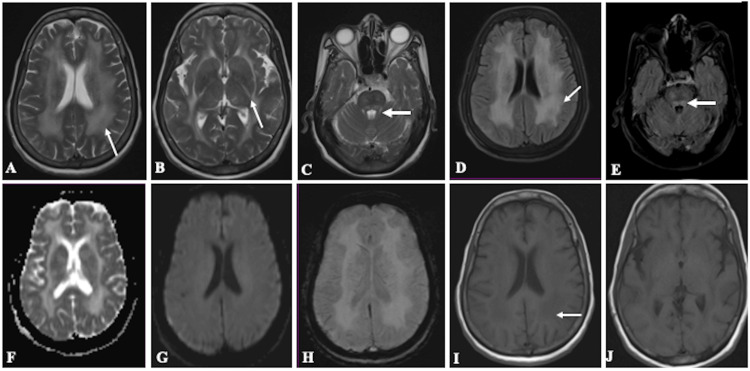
MRI of the brain images of the patient with lightning strike MRI: magnetic resonance imaging; T2W: T2-weighted; FLAIR: fluid-attenuated inversion recovery; ADC: apparent diffusion coefficient; SWI: susceptibility image Axial T2W (A-C) and FLAIR (D, E) images showed confluent symmetrical hyperintensities in subcortical, deep, and periventricular white matter of bilateral frontoparietal lobes, bilateral external and internal capsules (thin white arrows in A, B, D), and pons (thick white arrows in C, E). Axial ADC (F) and diffusion-weighted images (G) showed no restricted diffusion. Axial SWI (H) showed no evidence of blooming. Axial T1 images (I, J) showed that white matter lesions were mildly hypointense (thin white arrow in I)
